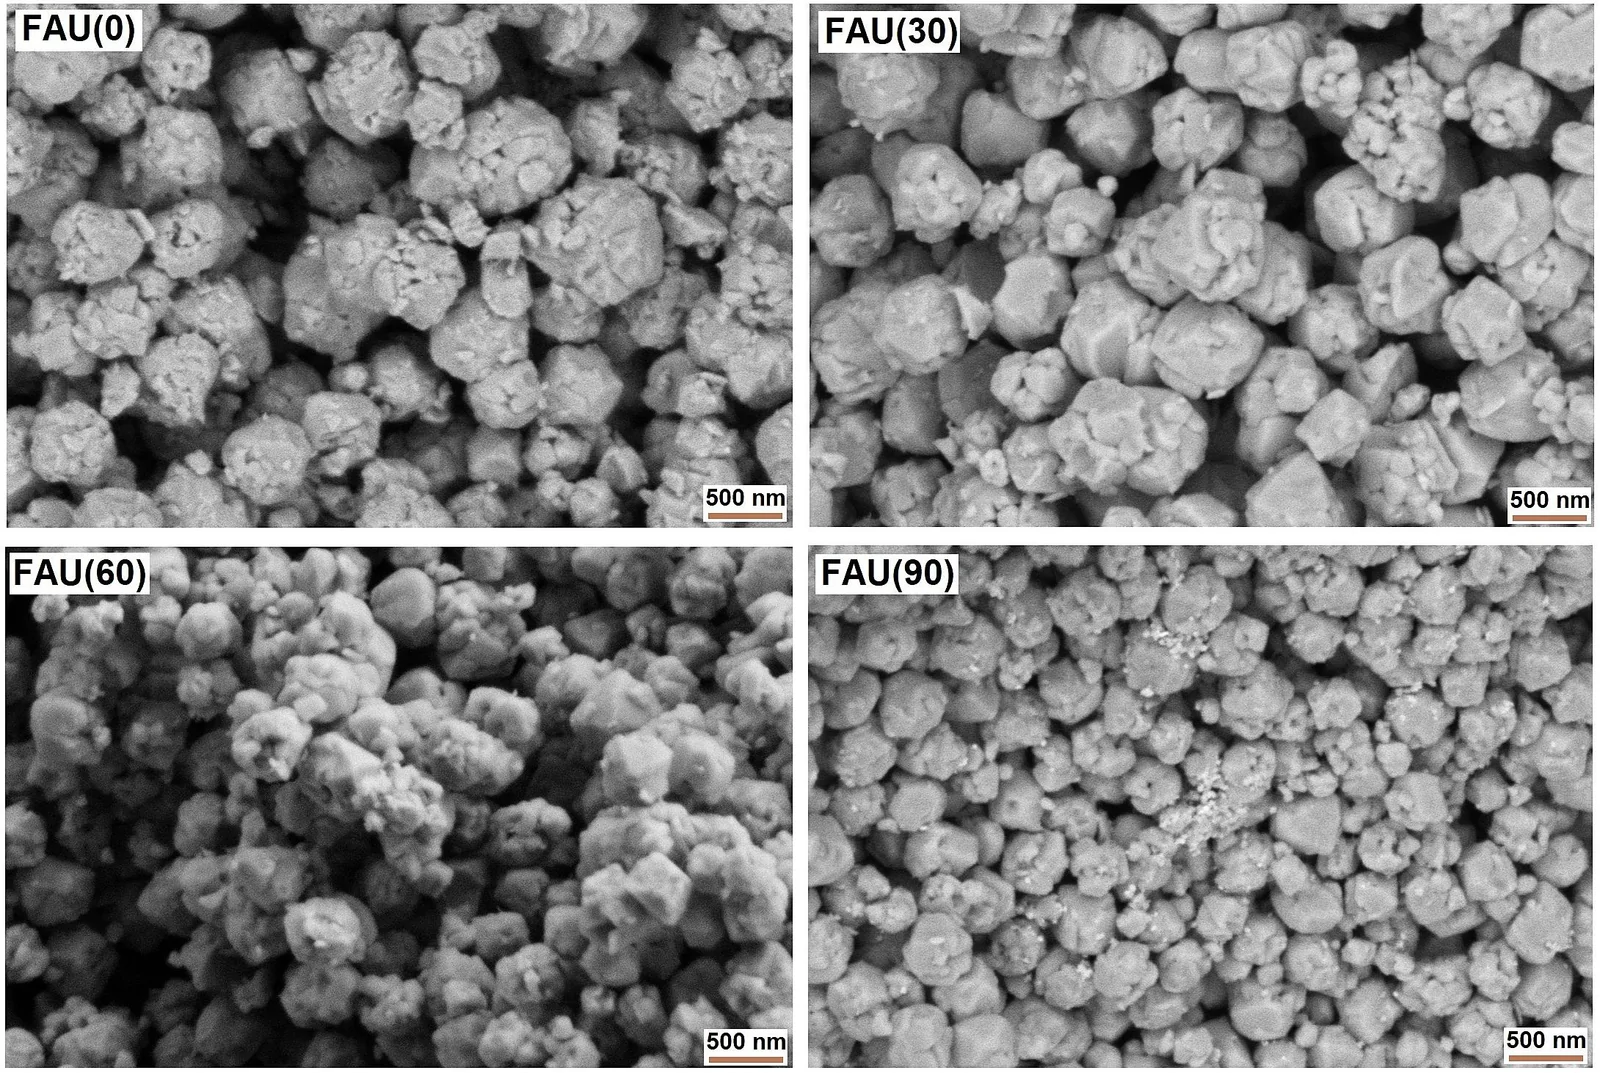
SEM images of the samples under study (magnification 80,000x). The influence of the exposure time of zeolite gel to ultrasonic irradiation on the morphology of the prepared zeolite systems.
